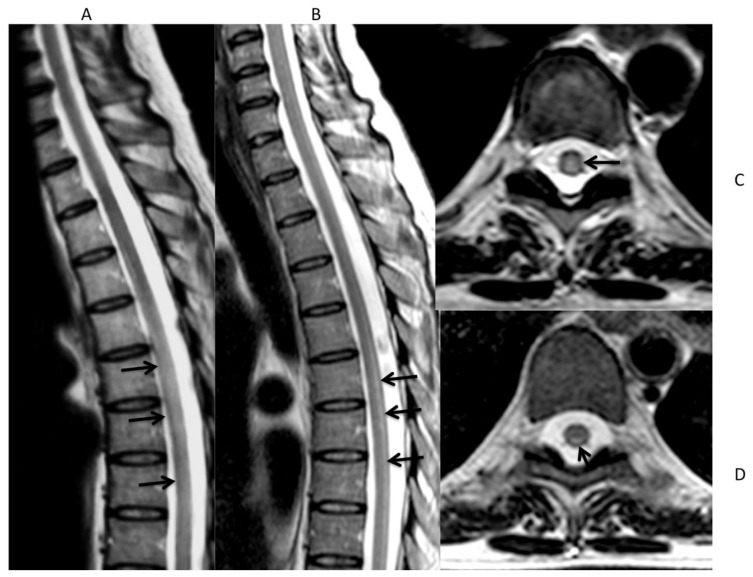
a,b,c,d. Sagittal and axial FSE T2w images at the onset of symptoms (a,c) and after 5 months (b,d). MR images obtained at the onset of symptoms show a diffuse hyperintensity of the dorsal spinal cord gray matter (arrows in a and c). After 5 months MRI shows only a linear residual hyperintensity involving the posterior spinal cord gray matter (arrows in b and d)
